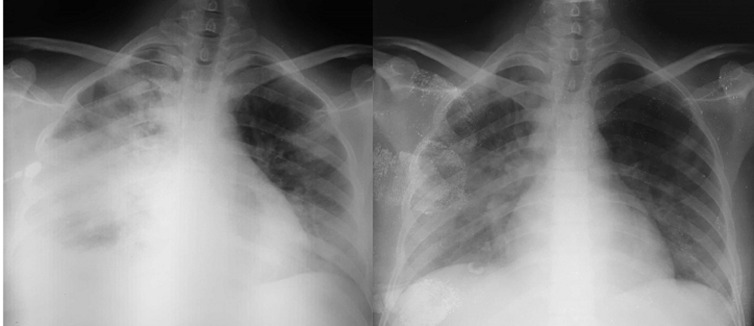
a chest X-ray on admission (on the left), compared to a chest X-ray on day 9 (on the right), showing a radiological improvement after nine days of treatment
